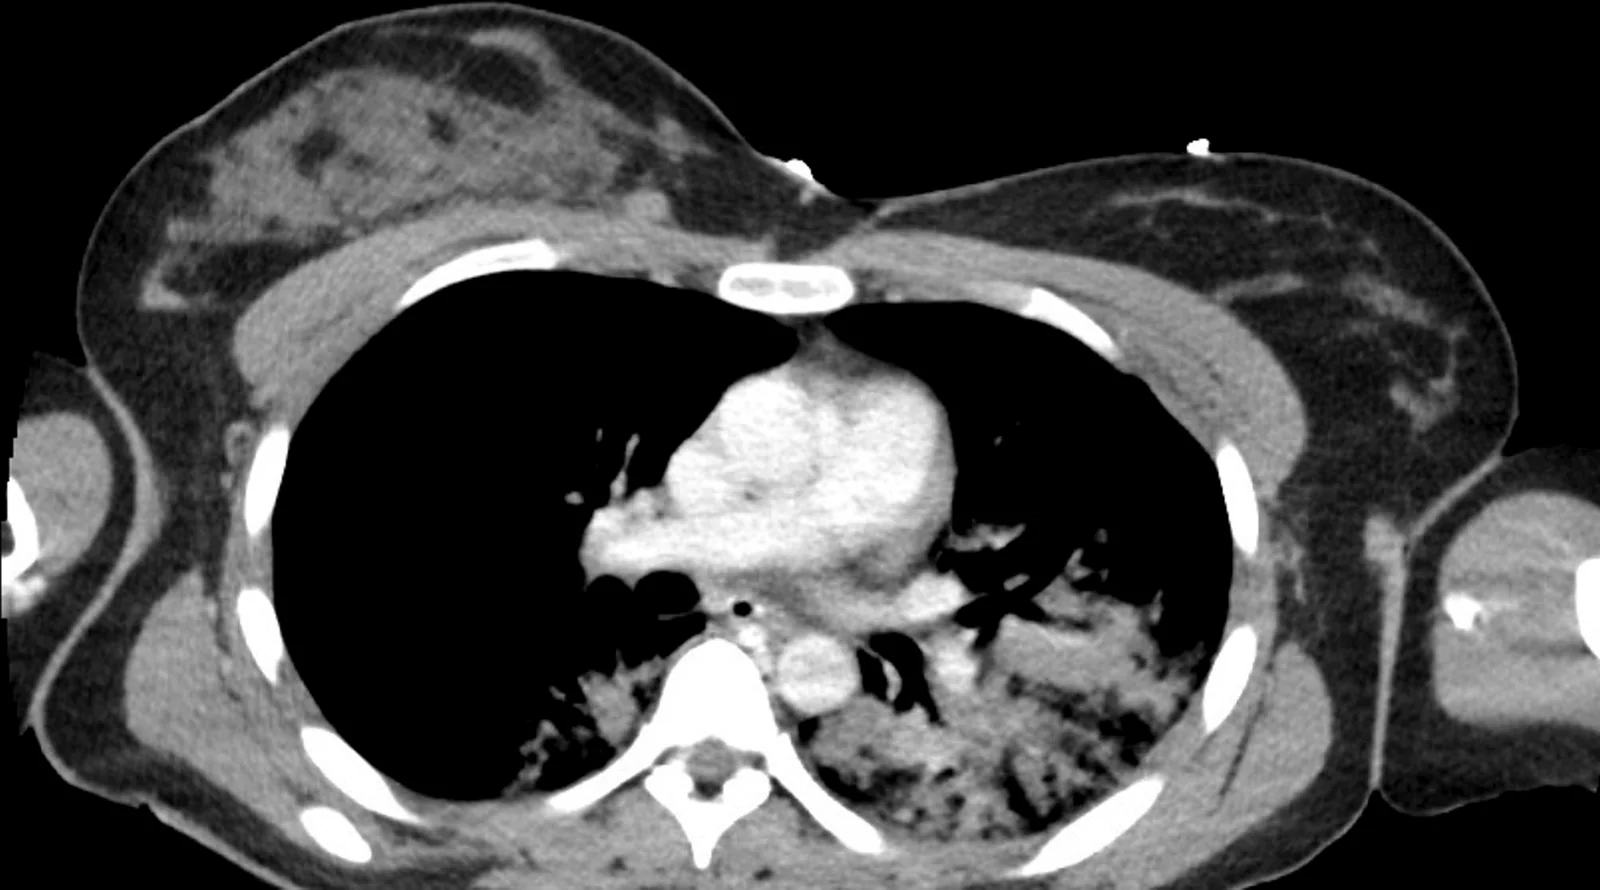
Chest CT pulmonary angiography Representative axial image showing bilateral dependent pulmonary opacities, more pronounced in the left lower lobe. No pulmonary arterial filling defect was identified on the complete examination.
